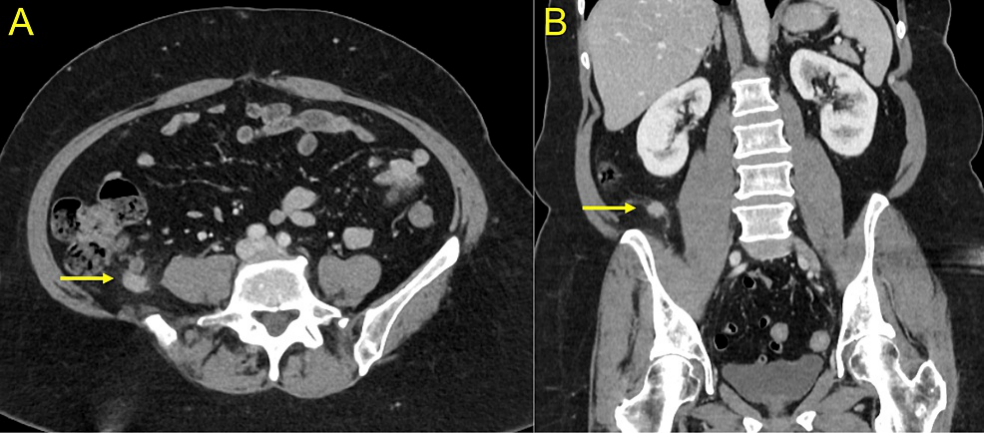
Seven-week follow-up computed tomography (CT) of the abdomen CT scan of the abdomen at the patient's seven-week follow-up visit revealed continued reduction in the size of the periappendiceal fluid collection (yellow arrows). (A) Axial view. (B) Coronal view.
